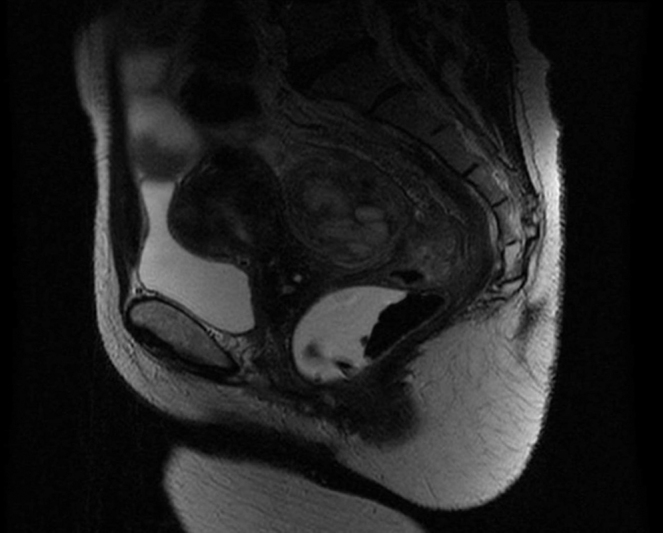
Sagital T2-weighted pelvic magnetic resonance image depicting the same large heterogeneous, mixed, retrouterine mass.
